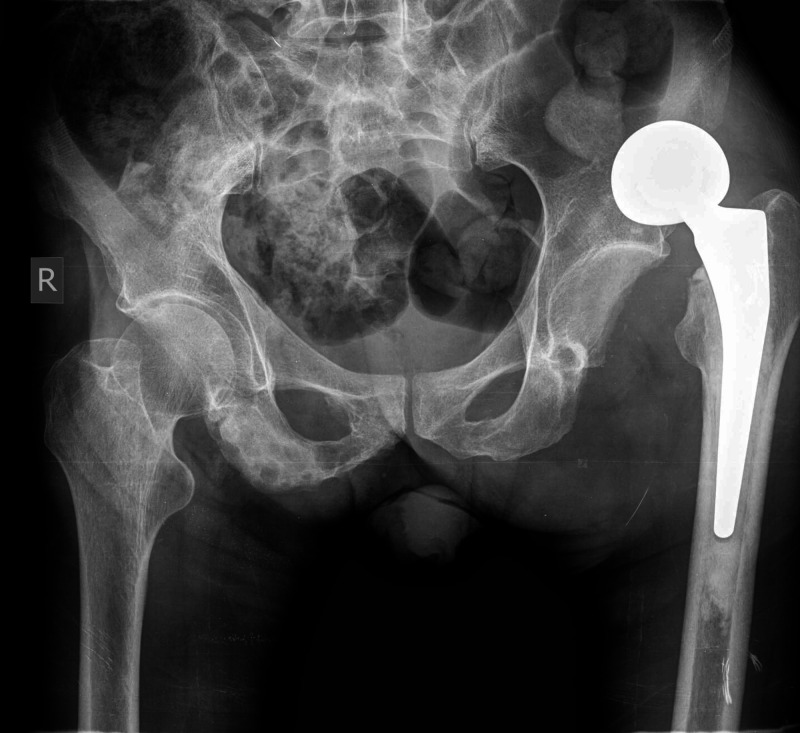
Postoperative anteroposterior pelvis radiograph demonstrating dislocation of bipolar hemiarthroplasty of the left hip
